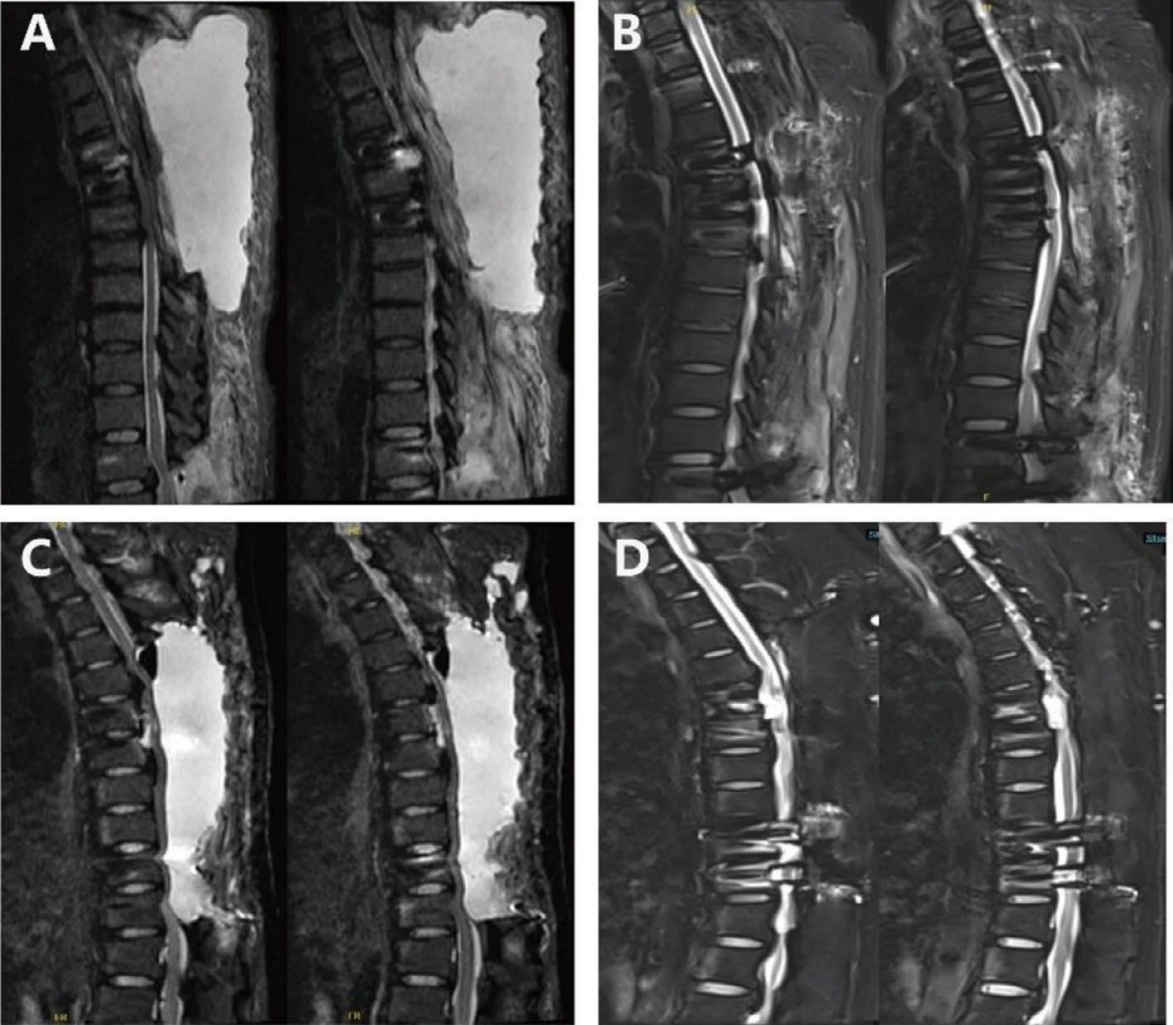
The MRI image (A) depicts the prepuncture condition of the patient in case1, wherein the high‐density region appearing as a bright white area represents PMC. Subsequently, (B) demonstrates a significant disappearance of the cyst after puncture. (C and D) show the patient in case2 before and after the puncture.
